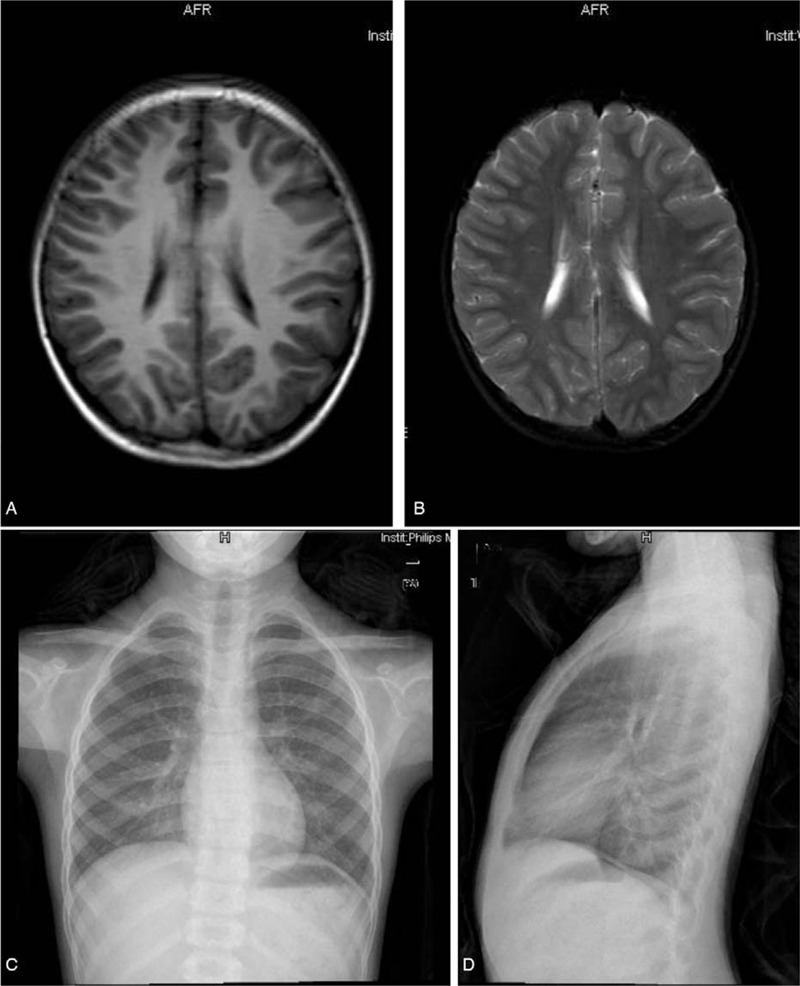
Axial T1-weighted and T2-weighted MR image of head (A-B). Frontal chest radiograph (C) and Lateral chest radiograph (D).
